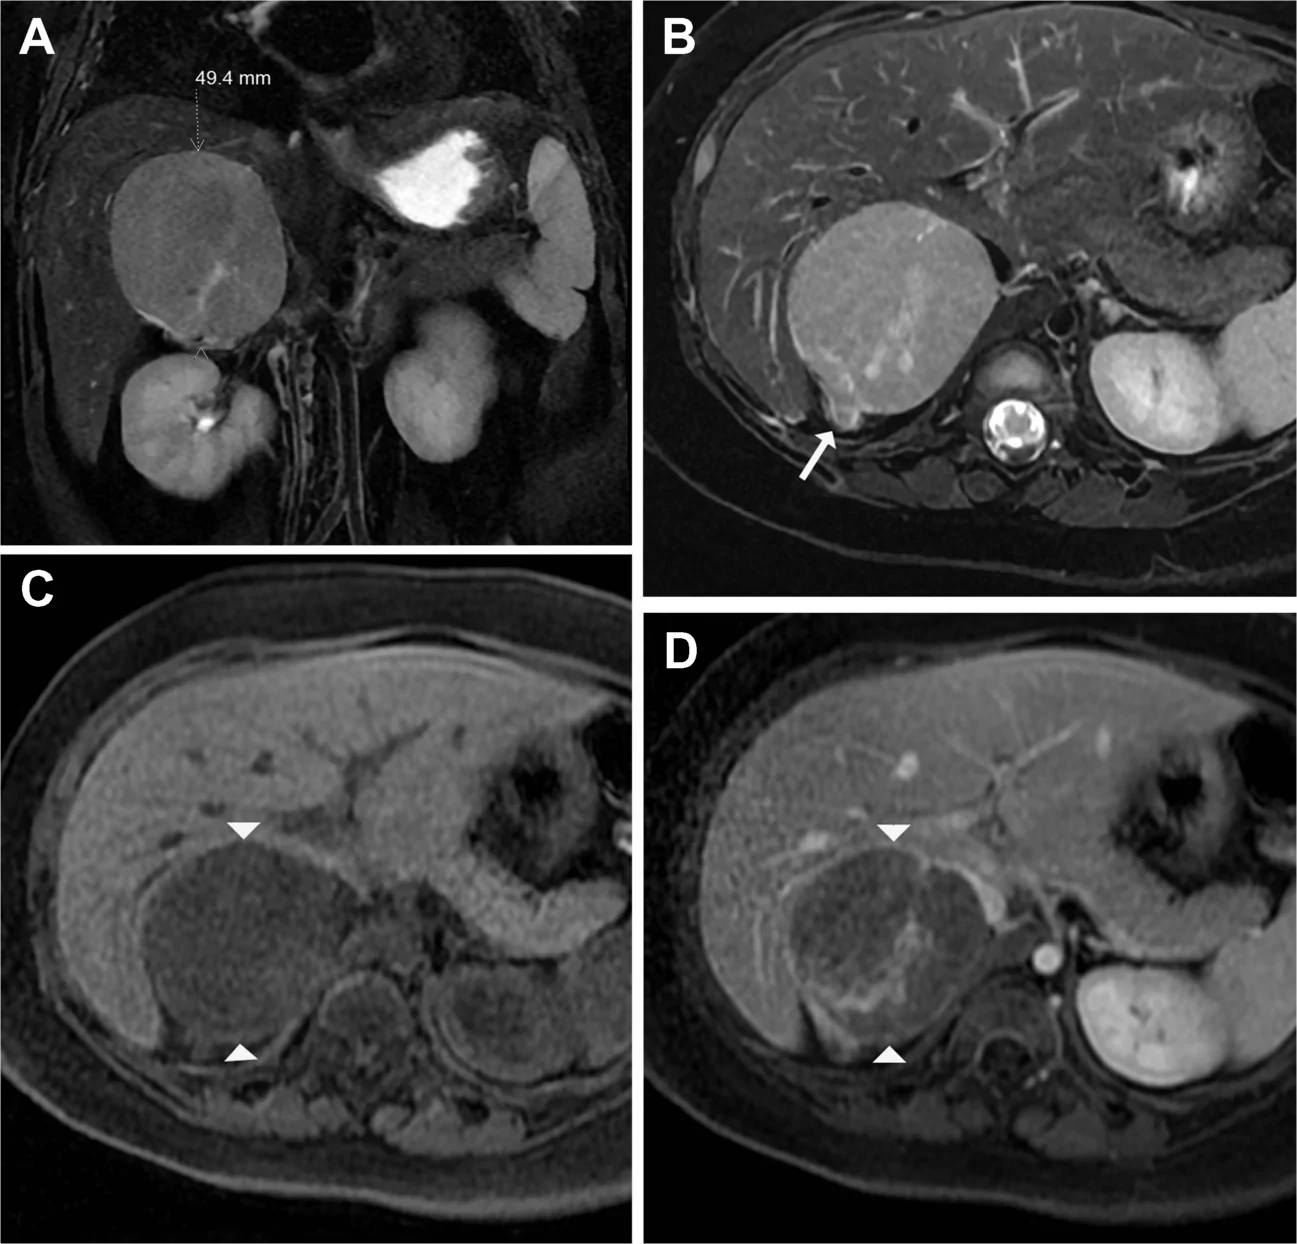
Adrenal cortical carcinoma in a 9-month-old female who presented with Cushing syndrome and hyperandrogenemia. a Coronal and (b) axial T2-weighted fat-suppressed images through the abdomen show a well-demarcated mass maximally measuring 4.9 cm in diameter with some internal heterogeneity. Of note, at the posterior margin of the mass, there is a small lobule of tissue extension with trace adjacent fat-stranding consistent with extracapsular extension (*arrow*), a finding concerning for aggressive tumor behavior and carcinoma. c Axial pre-contrast and (d) post-contrast T1-weighted fat-saturated images show rim enhancement and minimal internal linear and nodular enhancement (*arrowheads* indicate mass margins)
